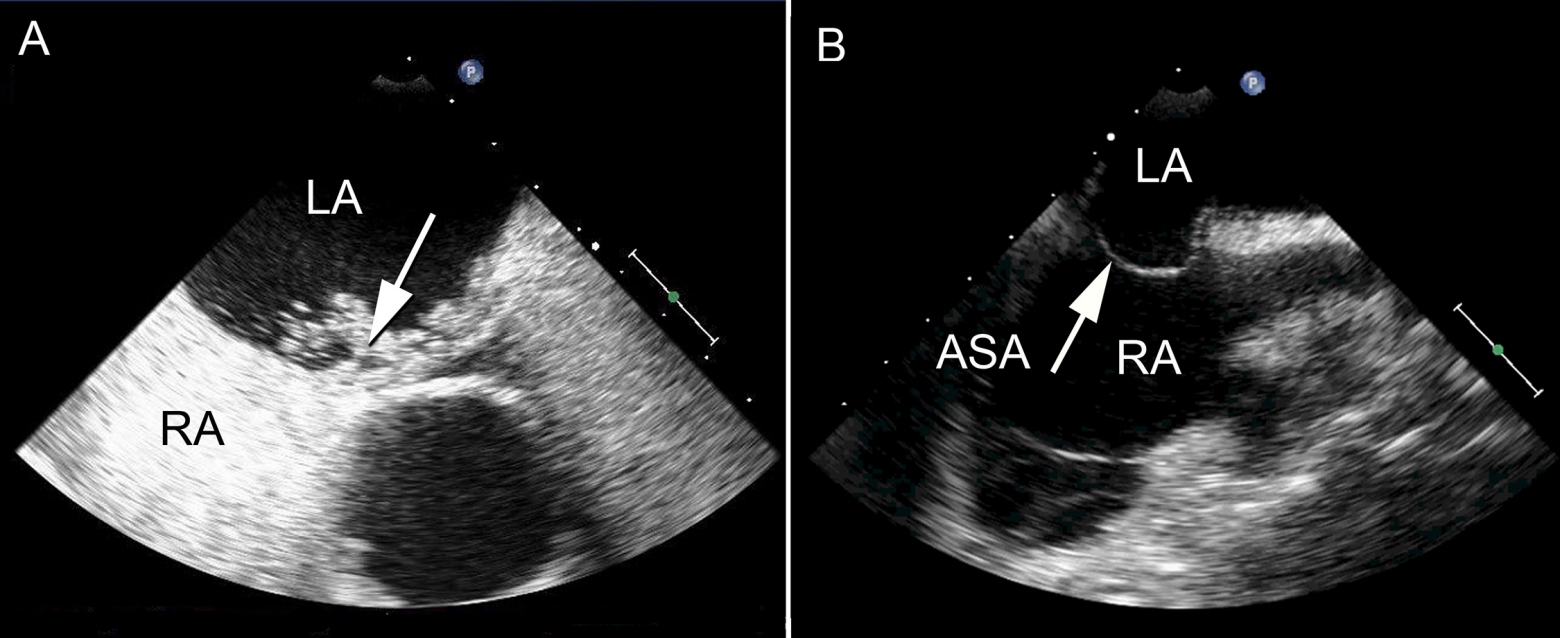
(A) Pre-procedural transesophageal echocardiography showing a medium-sized PFO (arrow) based on the extent of right-to-left shunt of agitated colloidal contrast. (B) Transesophageal echocardiography of a patient with a PFO and an atrial septal aneurysm (ASA; excursion of the septum of more than 15mm throughout the cardiorespiratory cycle). LA = left atrium; RA = right atrium.
